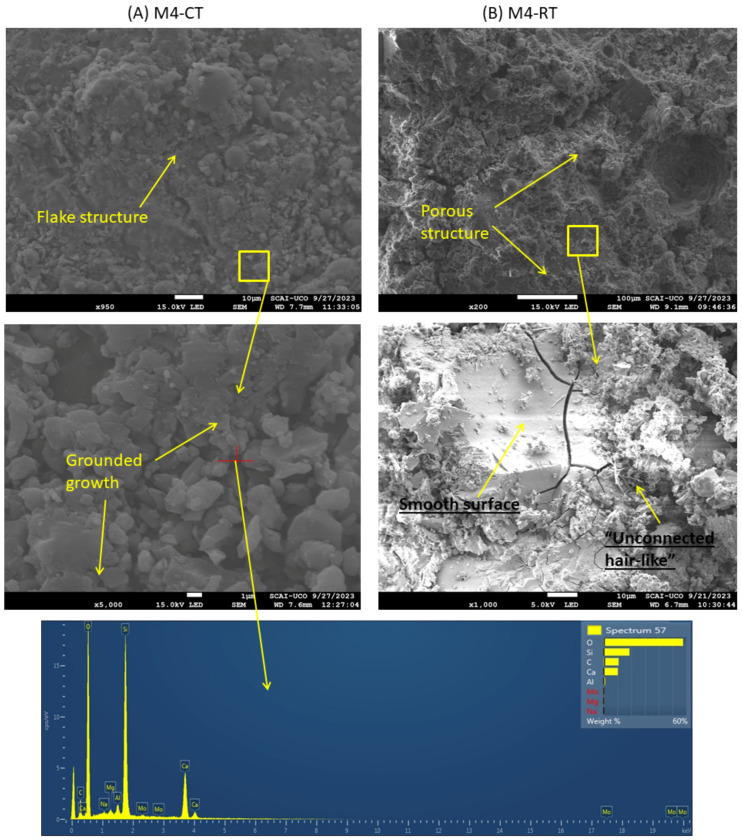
SEM and EDS of M4 under CT (A) and M4 under RT (B): the detected C originates from the alkali-activated material derived from coal fly ash and corresponds to the influence of the research method, not to the intrinsic composition of the sample.
